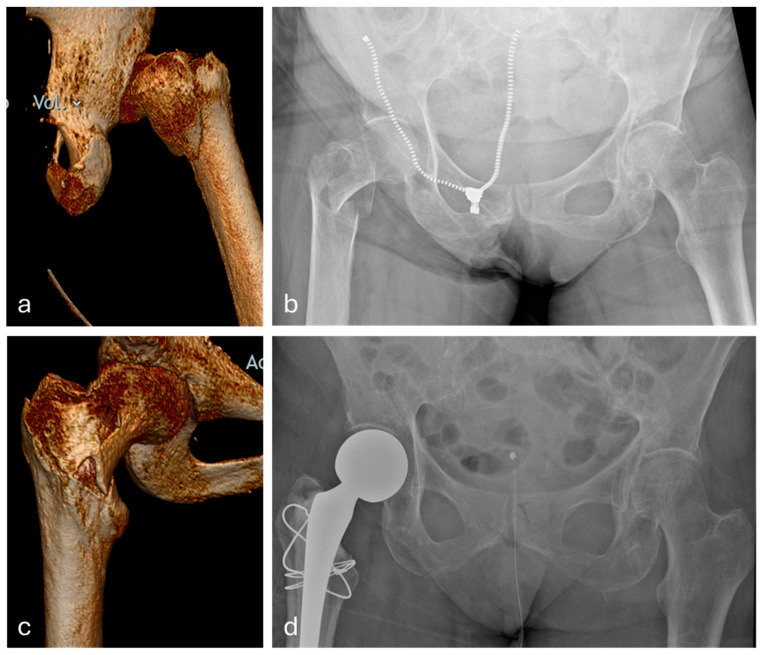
Pre and post op radiological images from a 77-year-old female patient whose abductor reconstruction was visualised in Figure 1. Three-dimensional CT visualisation of the right intertrochanteric neck of femur fracture (a,c). Pelvic radiograph demonstrating the same right-sided neck of femur fracture on admission (b). Pelvic radiograph post hemiarthroplasty and abductor reconstruction (d).
